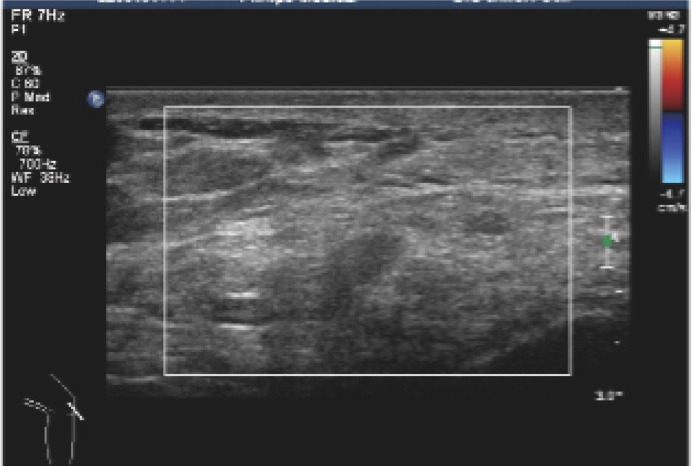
The ultrasound revealed a thickened layer of the skin and a subcutaneous layer of the left knee, with uneven and slightly strong echo and unclear boundary. The nodular hypoecho could be seen inside, and the internal blood flow signal was not rich.
